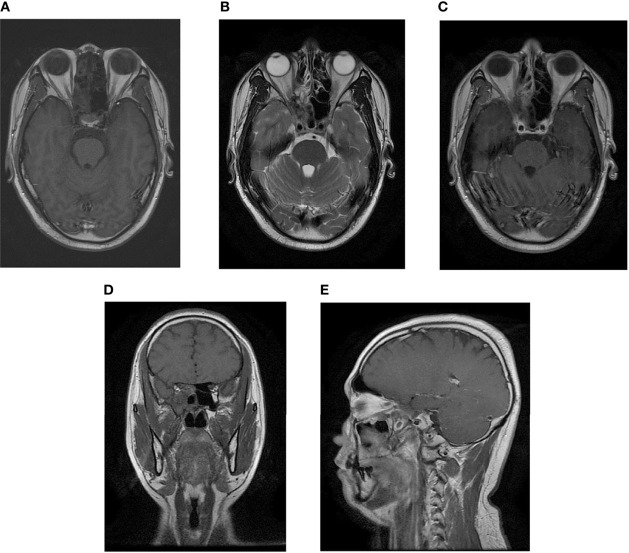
Postradiotherapy MR examination of the paranasal sinus. The MR examination detected a complete remission of the tumor. (A) Axial T1-weighted image. (B) Axial T2-weighted image. (C) Axial T1-weighted enhanced image. (D) Sagittal T1-weighted enhanced image. (E) Coronal T1-weighted enhanced image.
